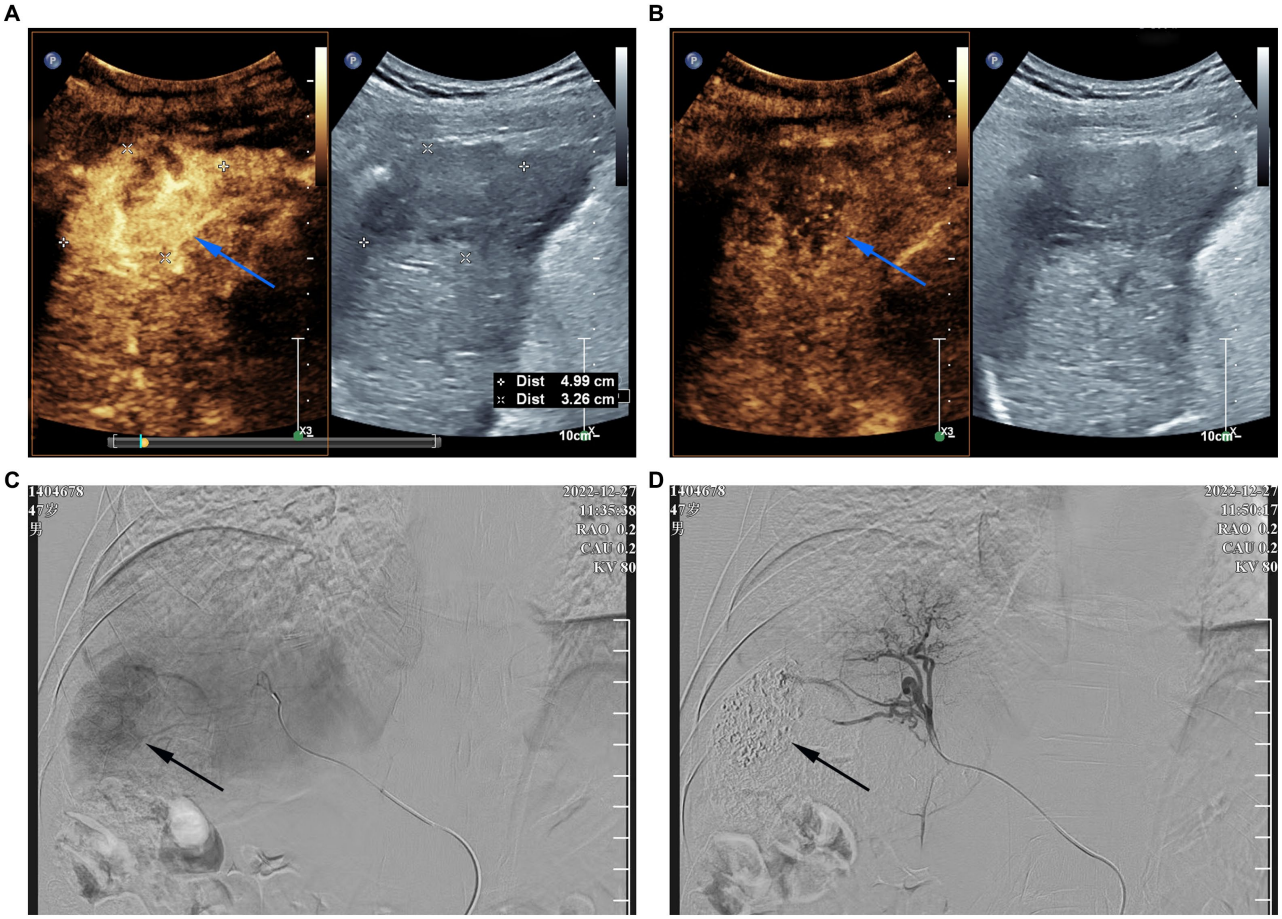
Contrast-enhanced ultrasound (CEUS) images and digital subtraction arteriogram (DSA) during TACE (A) CEUS in the arterial phase shows hyperenhancement of the mass [(A) arrow]; (B) The mass shows washout in the portal venous phase of CEUS [(B) arrow]; (C) pre-embolization DSA of the mass, black arrow represents tumor blush; (D) post-embolization DSA was repeated and demonstrated no residual enhancing tumor.
